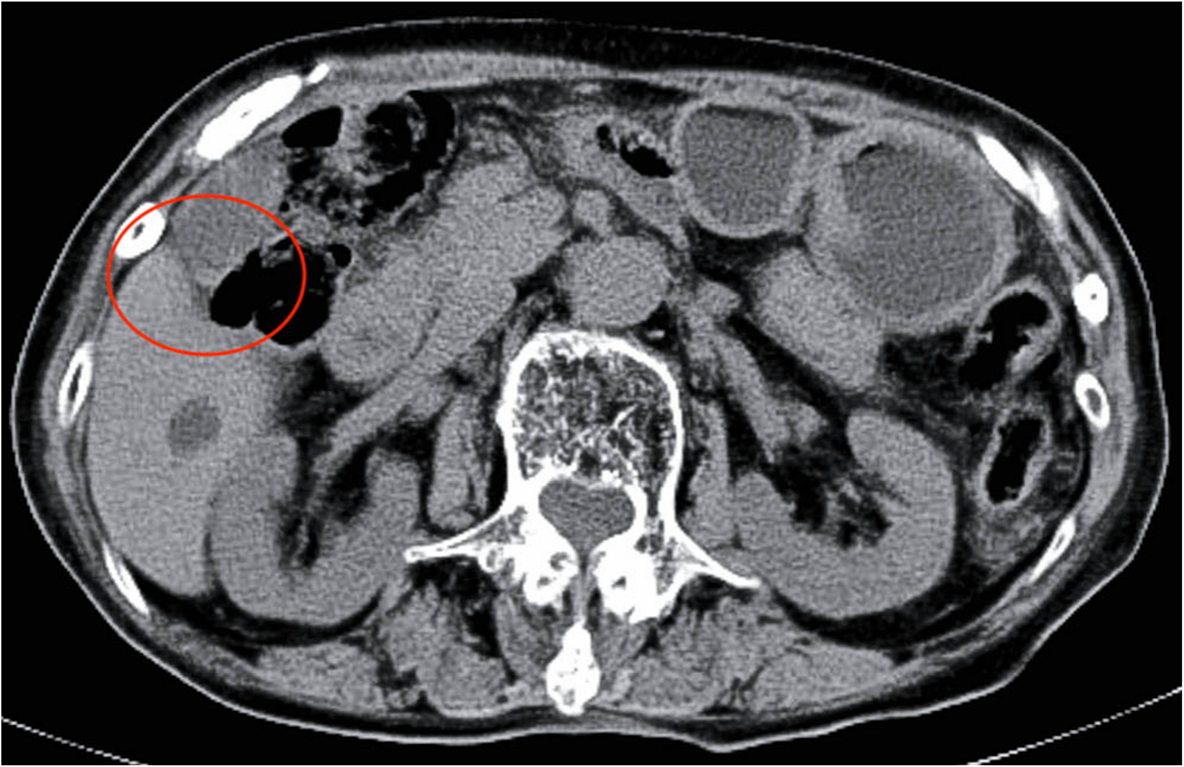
High-density image at gallbladder fundus in a computed tomography scan (*red circle*)
